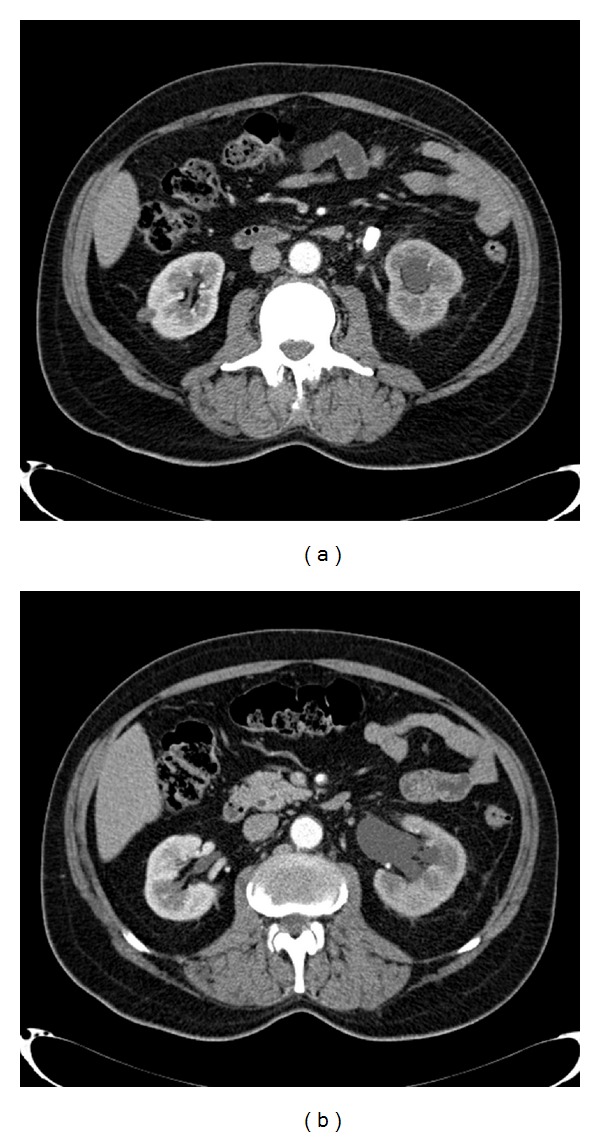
(a) Abdomen CT scan: left ureteral stone. (b) Abdomen CT scan: left hydronephrosis.
